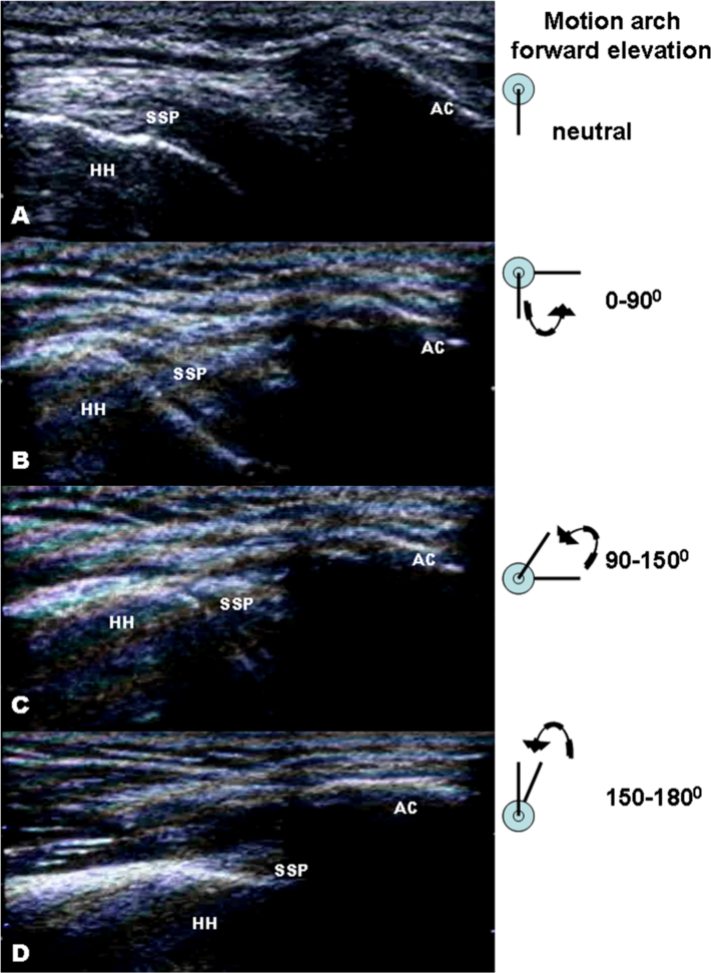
Sonographic findings without shoulder impingement. Dynamic musculoskeletal sonography of a 26-year-old man presented with no clinical impingement sign before implant removal. (A–D) Smooth passage of the supraspinatus (SSP) tendon under the acromion was observed while the shoulder was passively elevated from neutral to 180° of forward elevation. HH, humeral head; AC, acromioclavicular joint.
